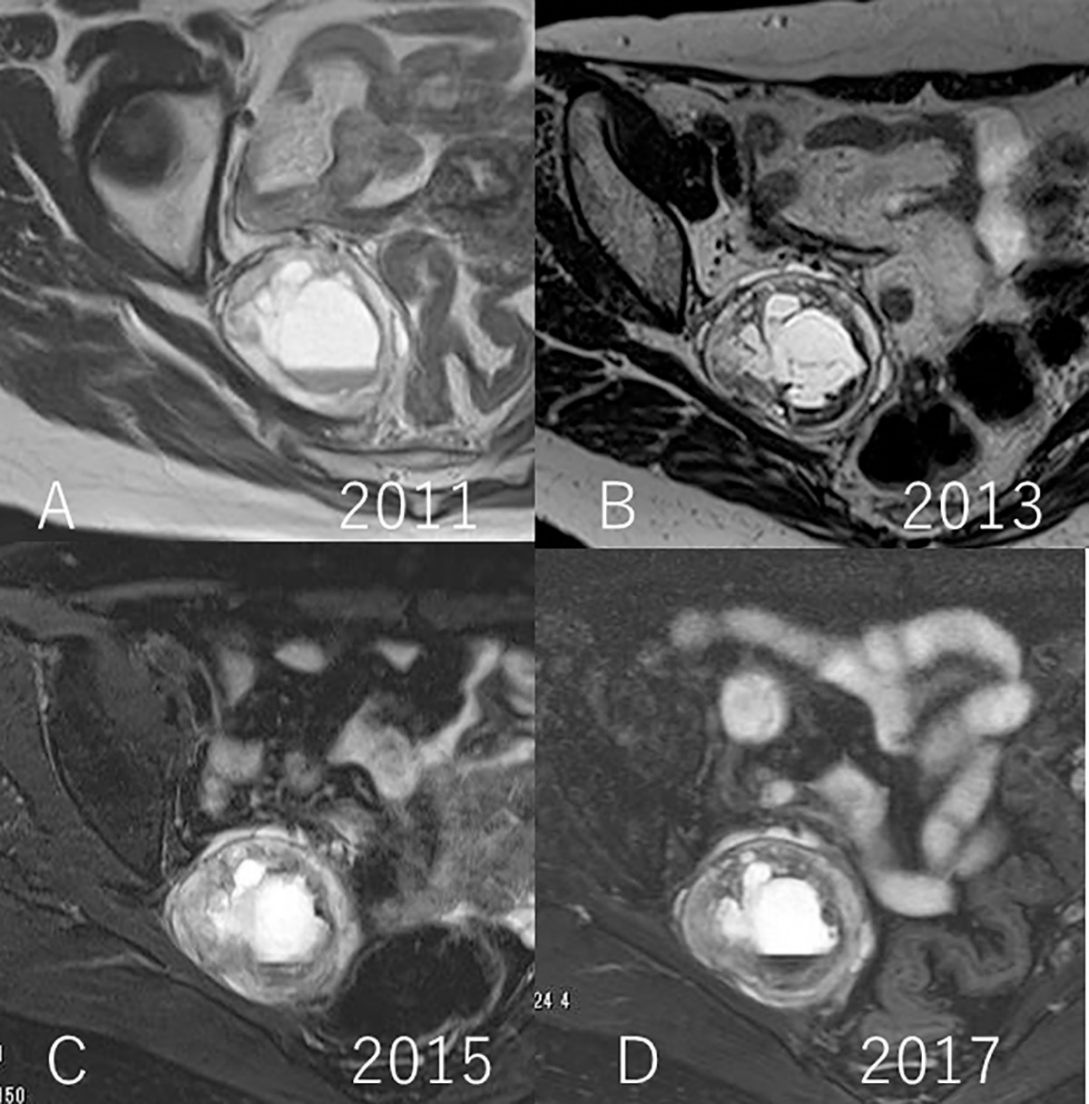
A. Initial T2 weighted MR image in 2011; B. T2 weighted MR image in 2013; C. T2 weighted MR image in 2015; D. T2 weighted MR image in 2017 (Case no. 4). These images show cystic change. Base line diameter of the tumor was 58 mm, and final diameter of the tumor was 54 mm.
